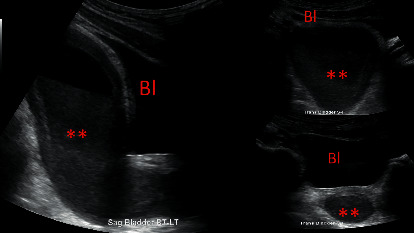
Our patient's ultrasound shows an enlarged fluid-filled structure (^*∗∗*^) filled with debris which is located in the midline and posterior to the bladder (Bl).
